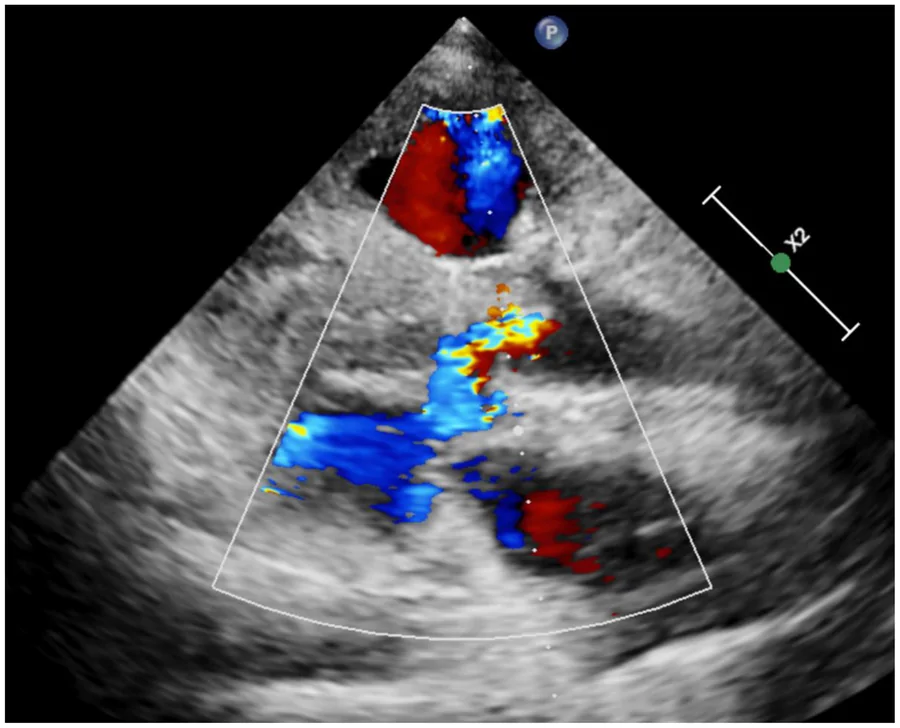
Postoperative color Doppler echocardiography demonstrating trivial residual aortic regurgitation following Yacoub remodeling valve-sparing aortic root replacement.
